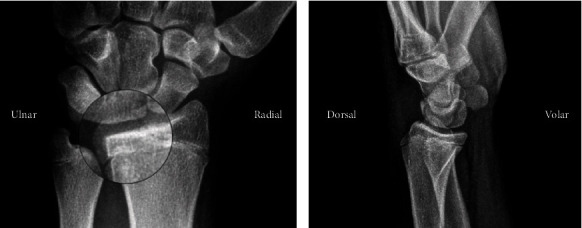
Plain PA and lateral radiographs of the left wrist demonstrating intra-articular fracture at dorsoulnar lip of the radius.
